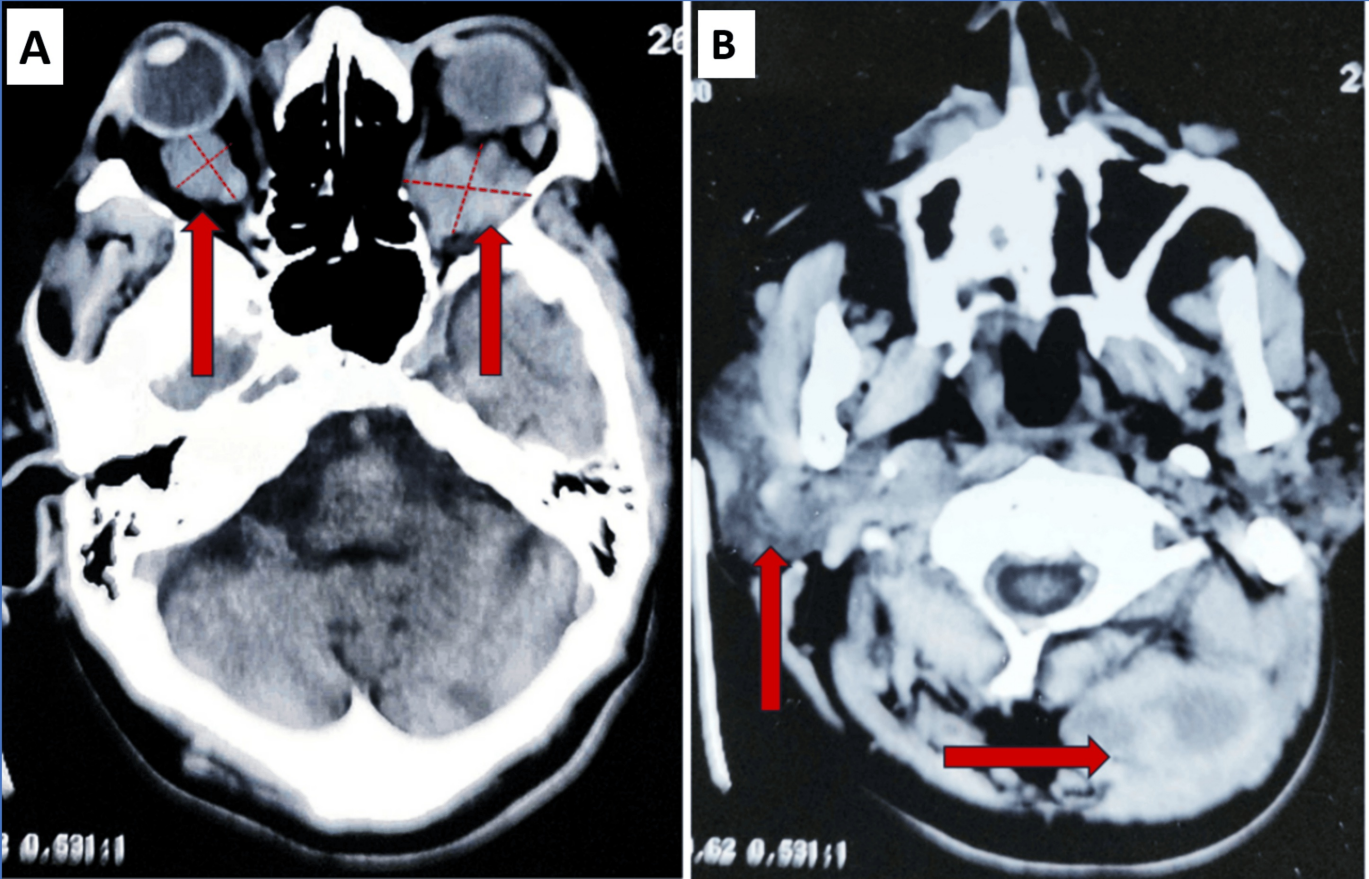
(A) Noncontrast CT head showing bilaterally asymmetric homogenous lobulated masses which is slightly hyperdense compared to extraocular muscles one on the right side and another retroorbital mass on the left side. (B) Some deposits of tumor in paraspinal muscles of neck evident as hypointense areas
